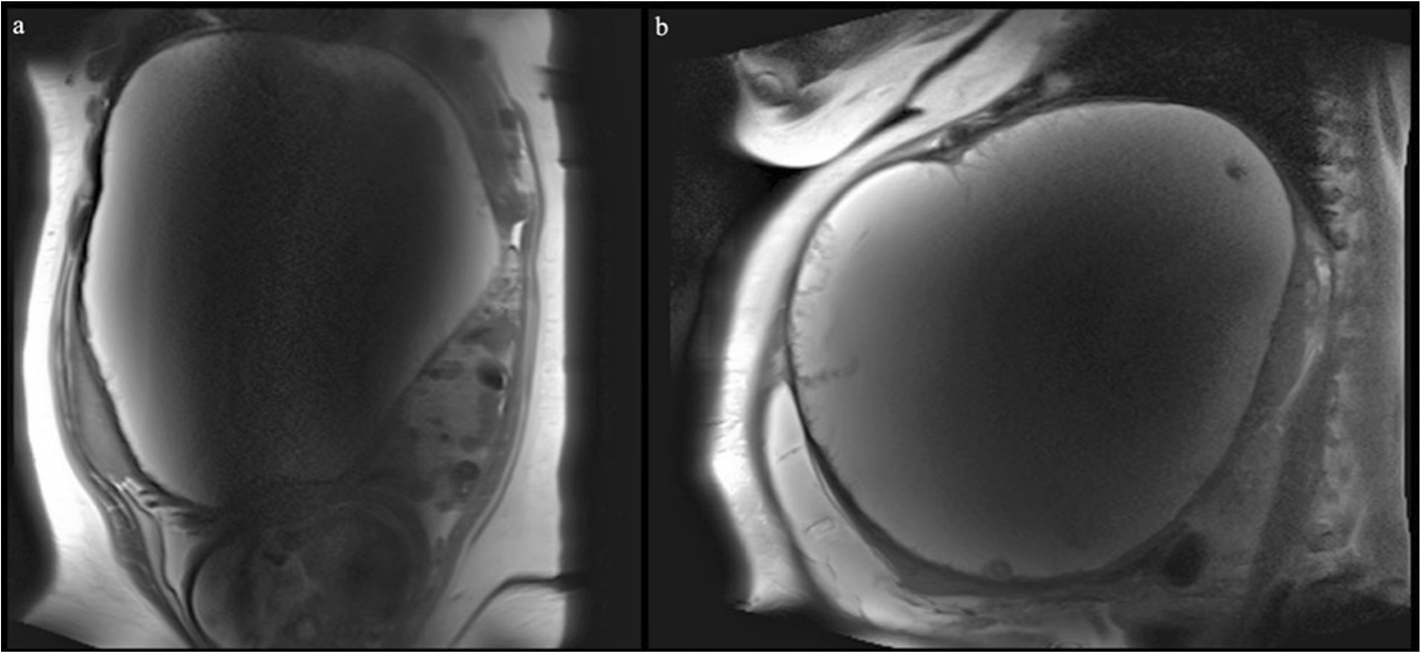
MRI of splenic cyst and fetus. a coronial view; b sagittal view
